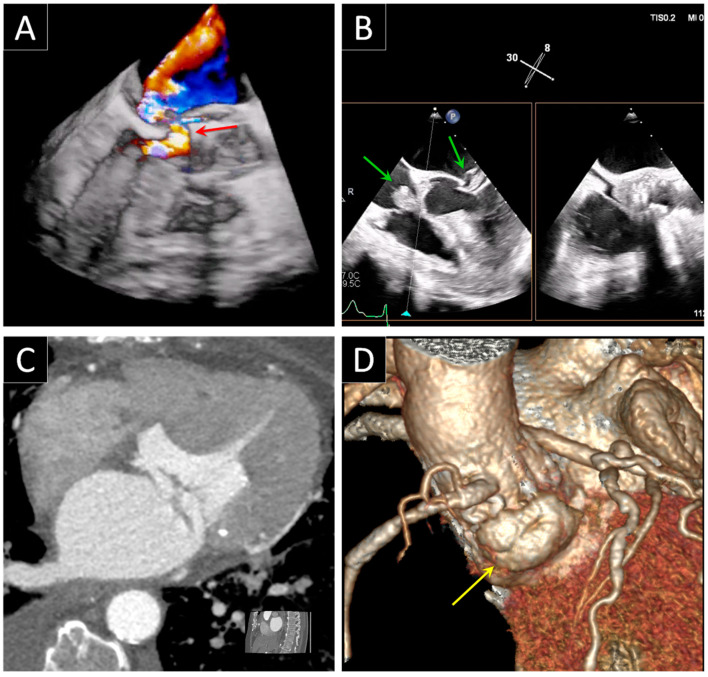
Bioprosthetic aortic valve abscess. (A) Standard 3D TEE merged with color demonstrating the presence of the PVL associated with the disruption of the mitral-aortic junction (red arrow) in a patient with infective endocarditis. (B) Vegetations are clearly visible on the tricuspid valve and mitral valve leaflets (green arrows) using 2D TEE x-plane view (C) CT image and (D) 3D CT reconstruction showing the abscess (yellow arrow) at the level of mitral-aortic junction.
